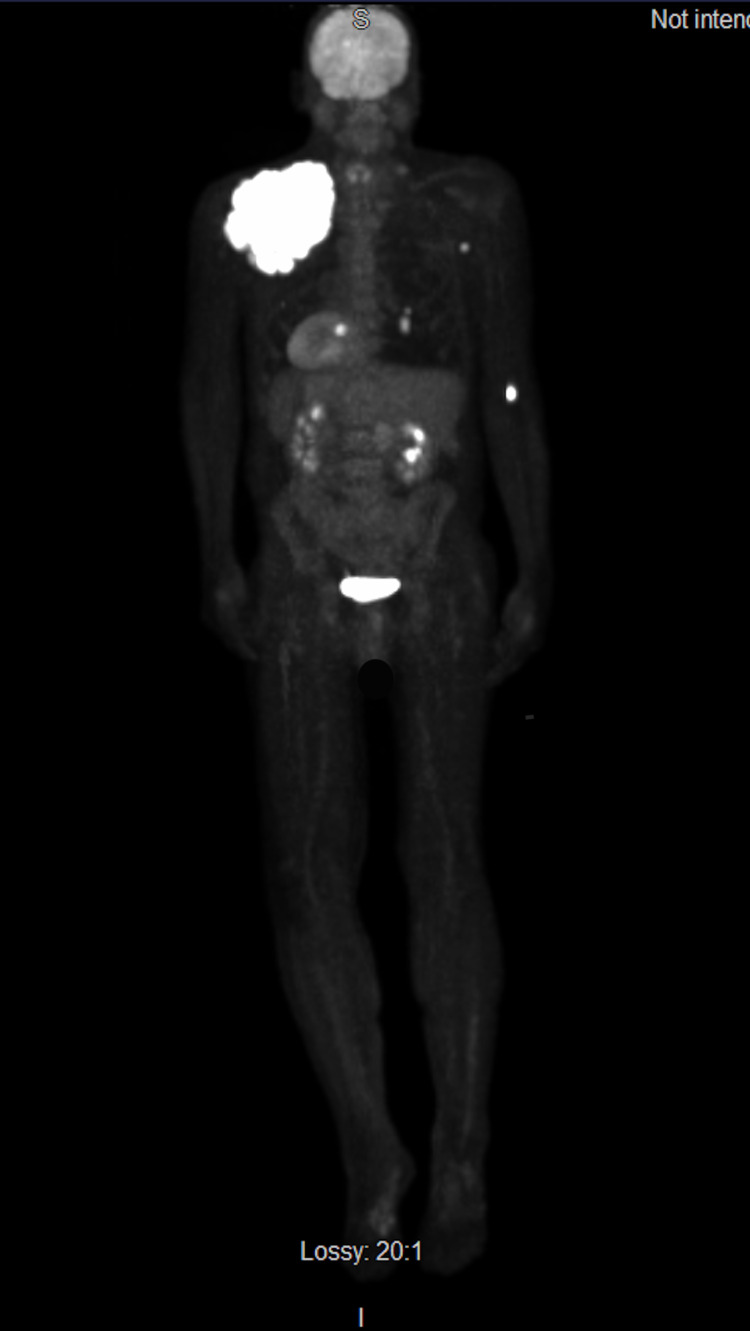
PET-CT displaying disease metastasis to the bilateral axillary lymph nodes, lung, and left plantar bone. PET-CT: positron emission tomography-computed tomography
